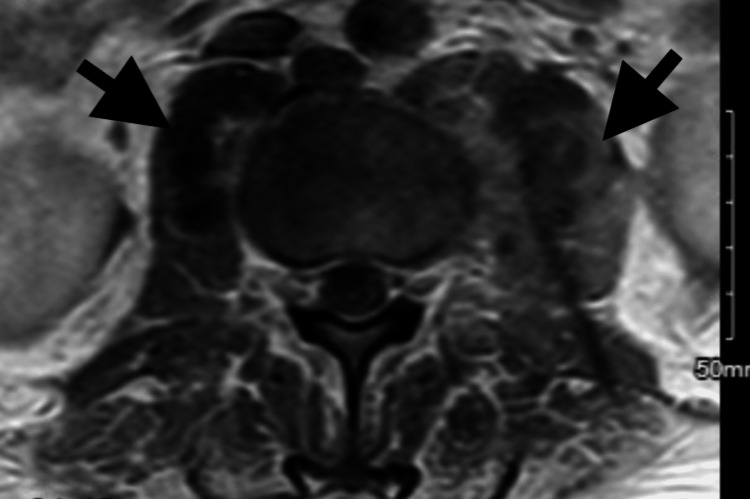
MRI with axial view showing bilateral psoas abscesses
